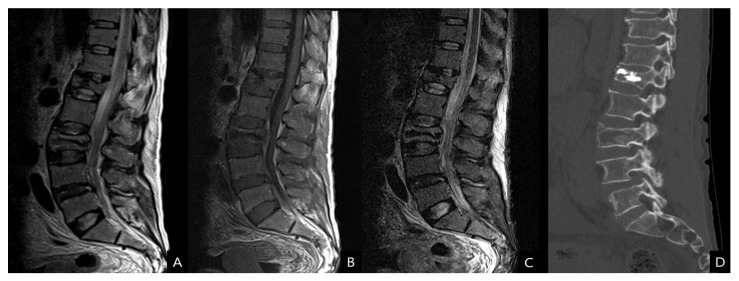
(A) T2-weighted, (B) T1-weighted, (C) short tau inversion recovery magnetic resonance images and also (D) computed tomography scan show a stable-type osteoporotic VCF at L3.
